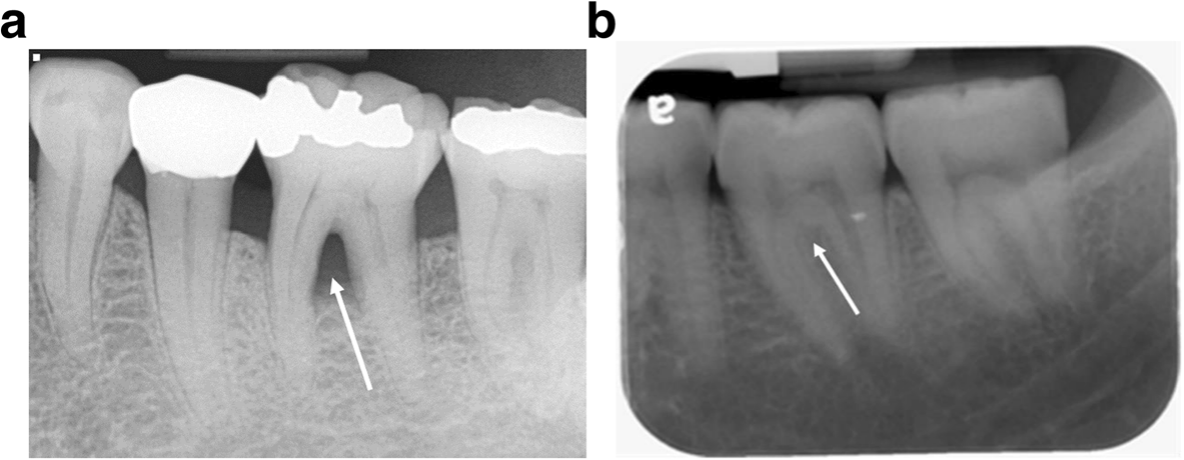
Intraoral radiographs demonstrate molar furcation status. a presence of furcation involvement. b absence of furcation involvement
